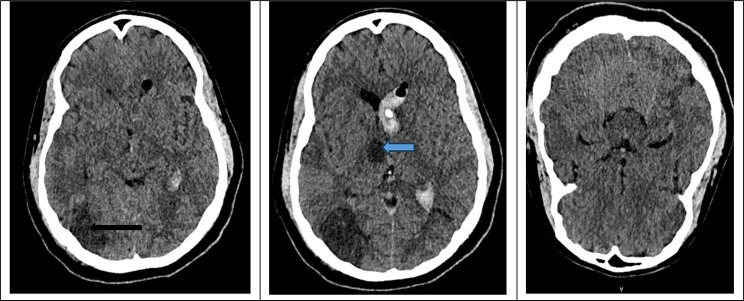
CT brain control done 5 days after 1st CT scan, showing new ischemic lesion in the left occipital region (black arrow) and in the left thalamus (blue arrow)
